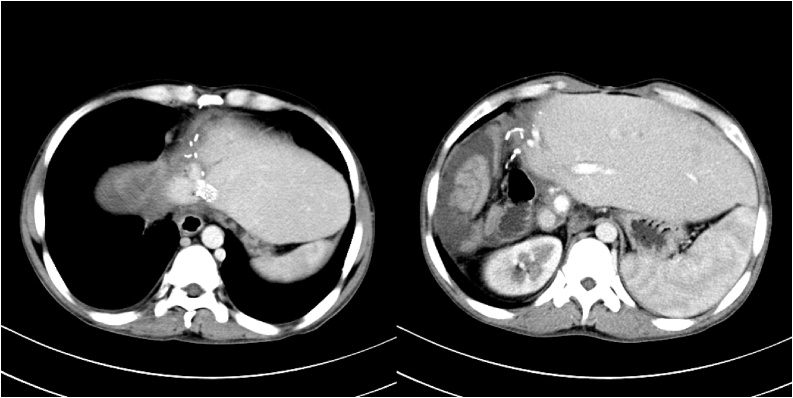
The CT scan indicated no recurrence, thrombus, liver congestion and cholangiectasis.
